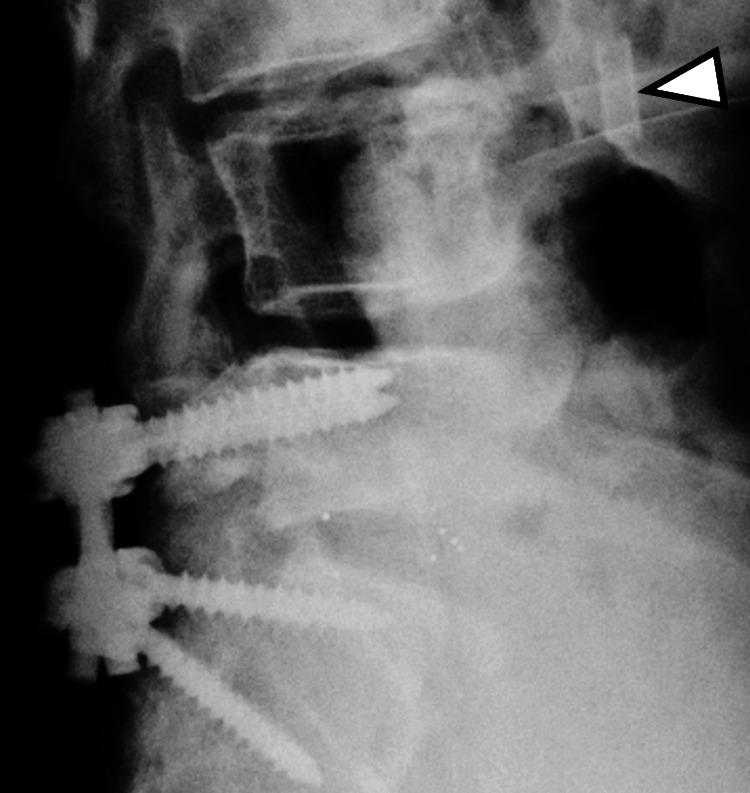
Immediate postoperative lumbar X-ray. A structure suggestive of the Nelaton cover is seen near the superior margin of the L4 vertebral body (white arrowhead).
